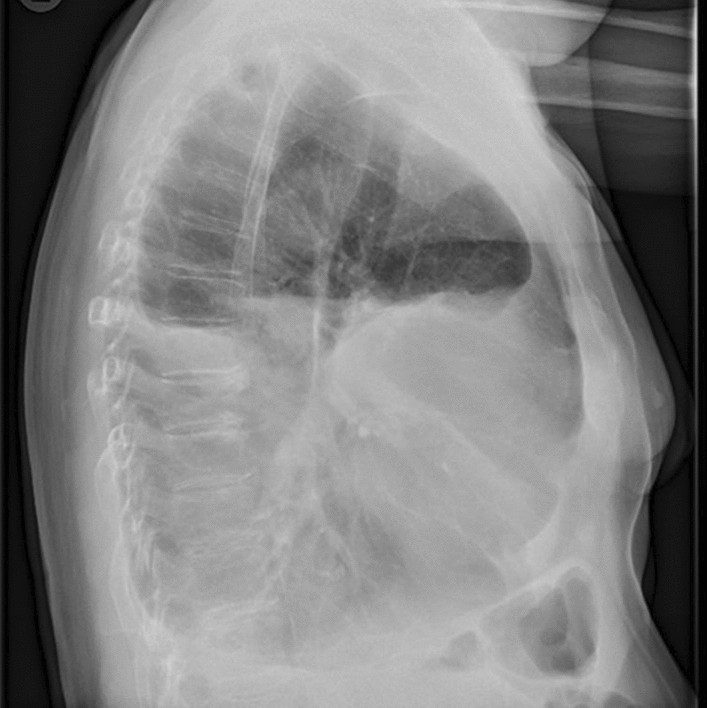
Chest X-ray—lateral view
